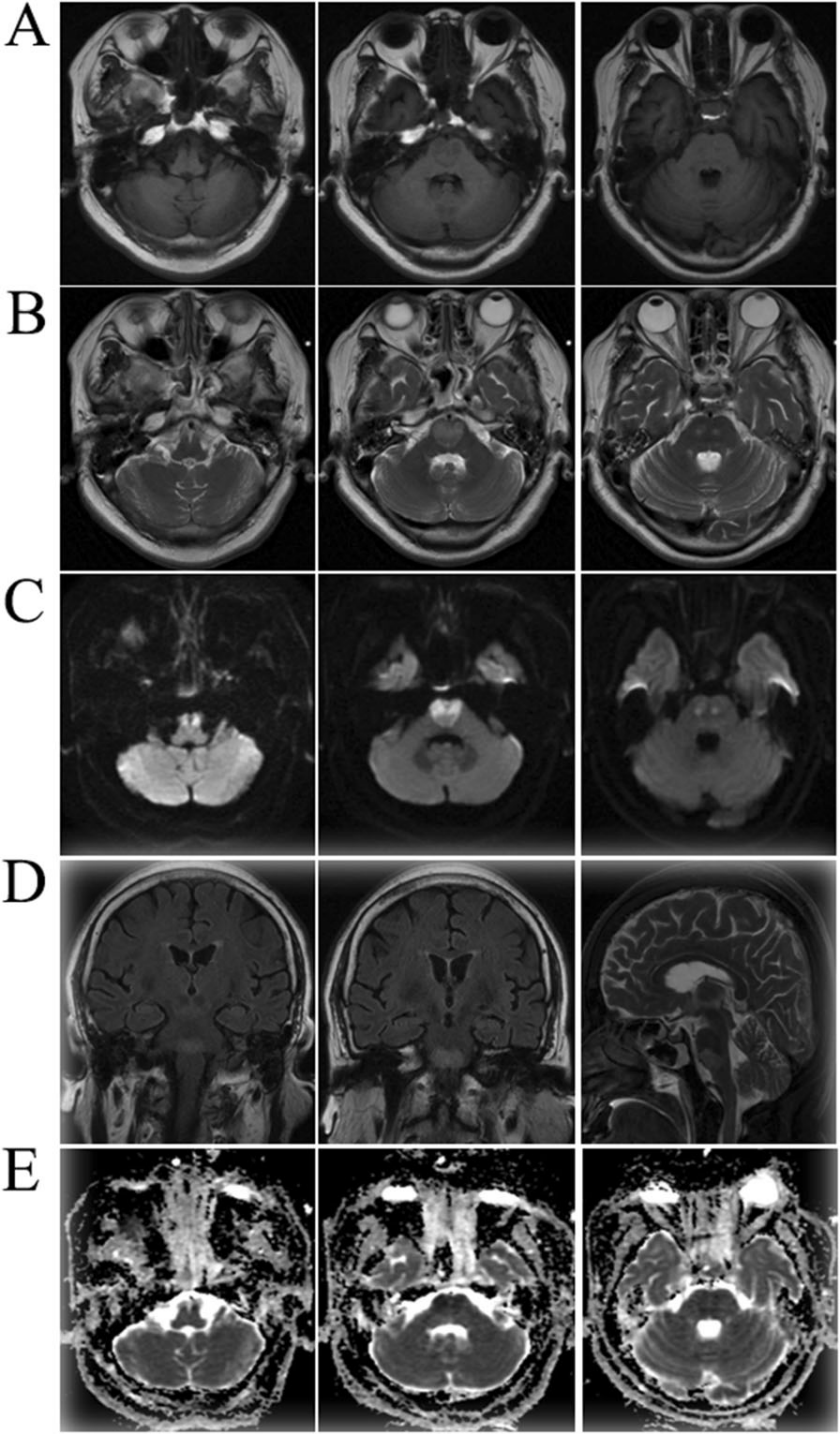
Cranial magnetic resonance imaging (MRI) indicated the giant “heart appearance” on the both sides in the ventral ponto-medullary junction. a, axial T1-MRI; b, axial T2-MRI; c, axial diffusion-weighted image MRI (DWI); d, coronal fluid attenuated inversion recovery (FLAIR) and Sagittal T2-MRI; e, axial apparent diffusion coefficient map MRI (ADC). The “heart appearance” lesion shows hypointensity on T1 and ADC, hyperintensity on T2, DWI and FLAIR, indicating, in conjunction with the finding on DWI, that the lesion is a subacute infarct
